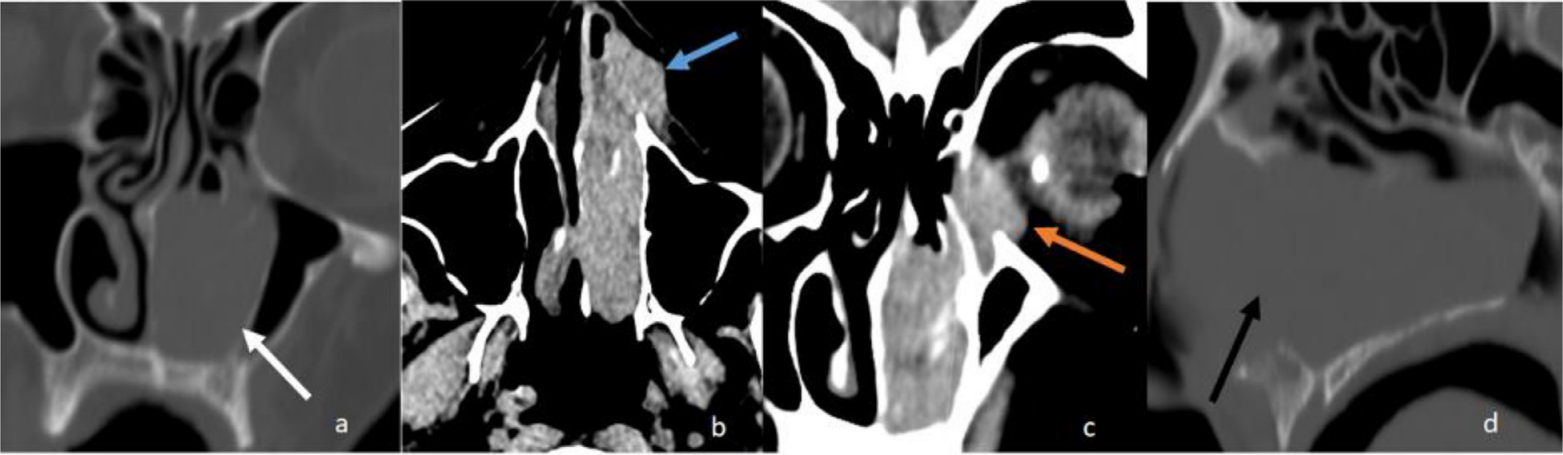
Paranasal sinus CT scan at presentation. (A) Coronal view bone window demonstrated mass centered in left nasal cavity with expansion of the nasal cavity and involvement of the inferior turbinate (arrow), (B) Axial view soft tissue window demonstrates extension into the nasal aperture, (C) Extension into the left medial orbit extraconal space, (D) Sagittal view bone window demonstrates nasal cavity expansion with no bone destruction. Differential diagnosis at this stage included: sinonasal carcinoma (including adenocarcinoma and squamous cell carcinoma), sinonasal granulomatosis with polyangiitis, and sinonasal melanoma.
